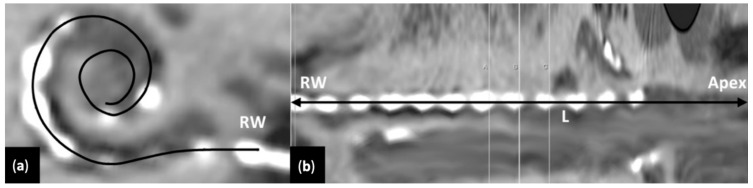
Postoperative CT scans analysis and reconstruction: (a) Oblique view of the cochlea on a multiplanar reconstruction (MPR) with minimal intensity projection showed the full length of the electrode array; (b) A reconstruction of the image (a) in a curvilinear plane unfolded the cochlear spiral; The relative distance of each electrode to the round window (RW) was measured. Since the electrodes are not on the same plane, they cannot be all visualized on the MPR.
